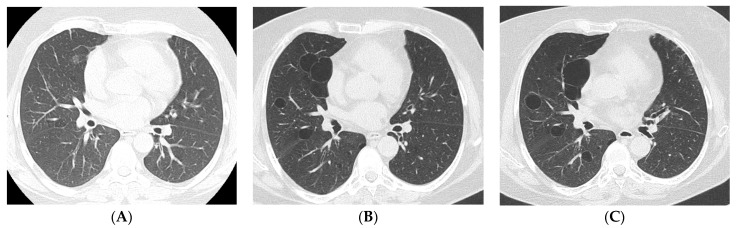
HRCT scan of a 42-year-old female pSS patient in 2008 (A) showing only very modest signs of bronchiolitis. She went on to experience recurrent episodes of acute dyspnea and cough, and follow-up HRCT scans in 2011 (B) and 2021 (C) document progressive formation of thin-walled cysts. The combination of these imaging findings and appropriate clinical context in a pSS patient is virtually pathognomonic of LIP. Of note, confluence of LIP cystic lesions into larger ones (as is shown in (C)) exposes the patient to higher risk of complications.
